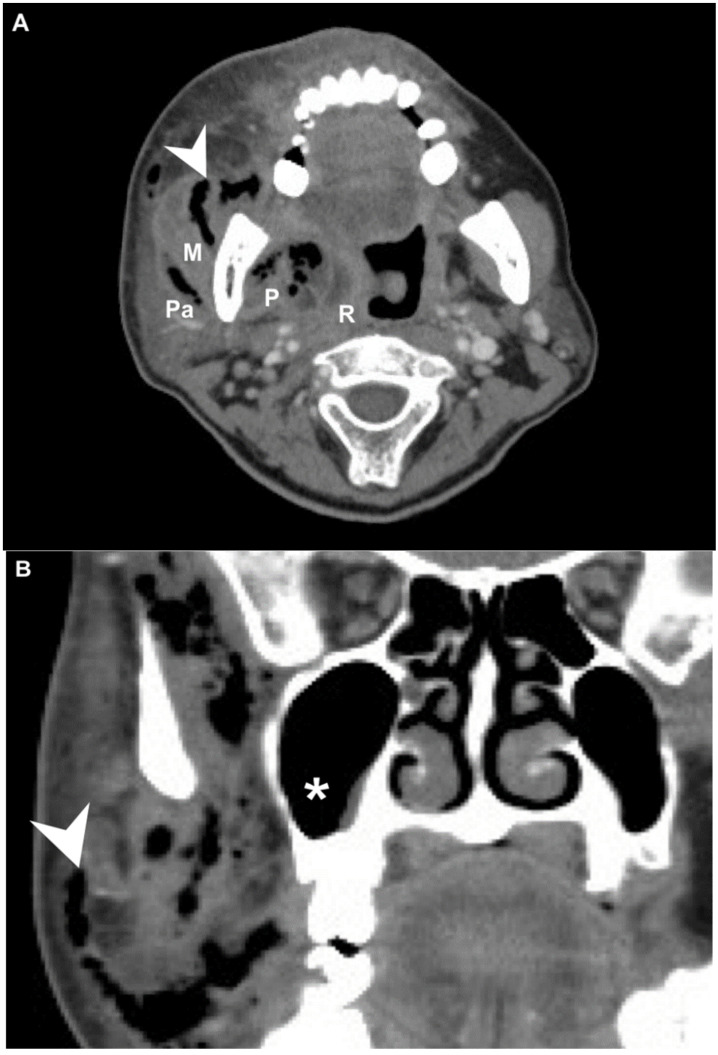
(A) Axial and (B) coronal view of a patient with concurrent deep neck infection and cervical necrotizing fasciitis on CT. Arrowhead: cervical necrotizing fasciitis; asterisk: maxillary sinus; P: parapharyngeal space; R: retropharyngeal space; M: masticator space; S: submandibular space.
